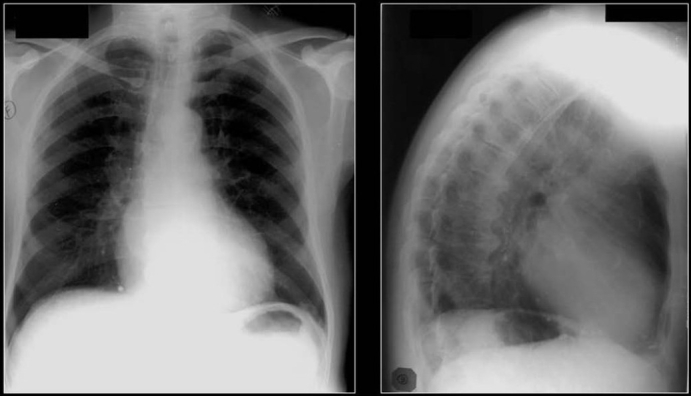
A chest radiograph shows thorotrast deposition in posterior mediastinal lymph nodes seen as tiny metallic nodules. This patient had a carotid angiogram performed in the mid forties for a subarachnoid hemorrhage where thorotrast was used as a contrast agent. The spleen, which is not clearly depicted here also showed lace-like metallic densities due to thorotrast deposition
